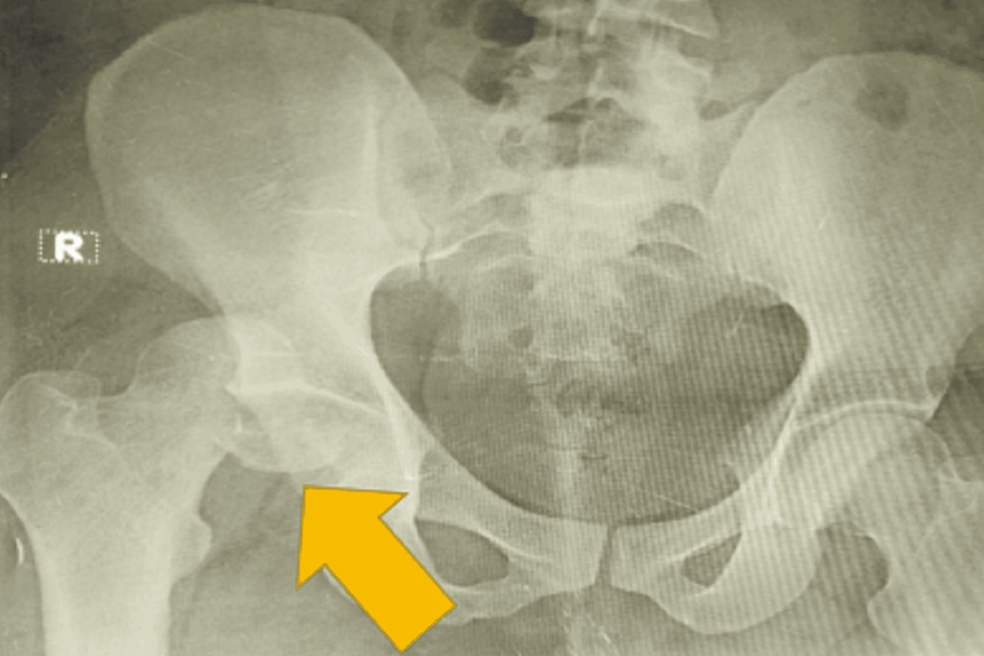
Plain radiograph of right hip showed fracture of head of right femur with posterior dislocation of right hip.
